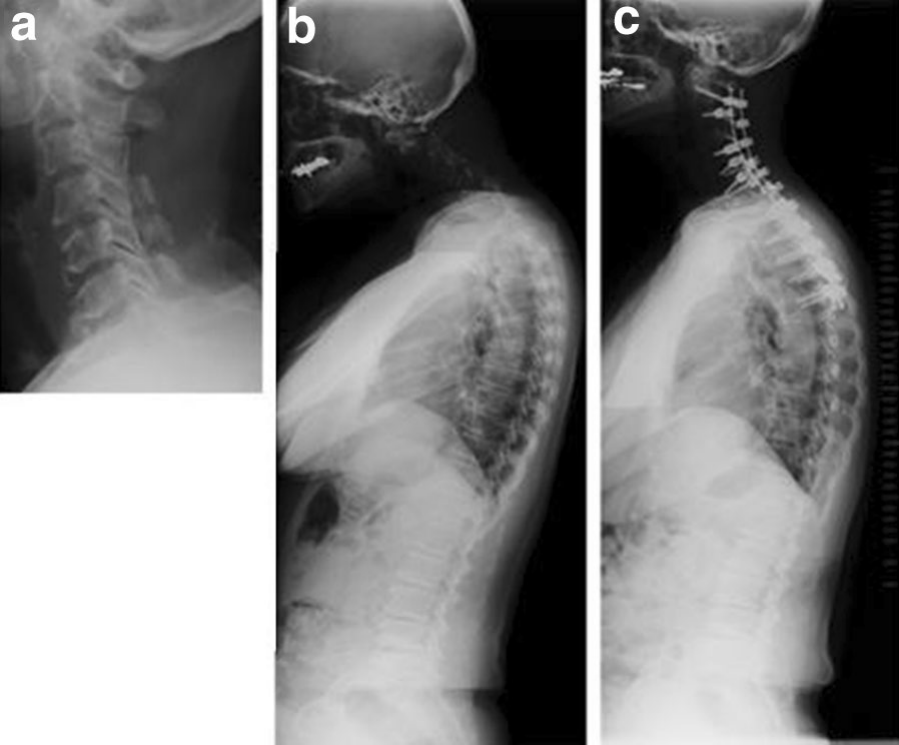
Case 1 radiographs. a Lateral plain radiograph of the cervical spine after laminoplasty for cervical spondylotic myelopathy showing normal sagittal alignment. The patient complained of head drop and severe low back and lower extremity pain 2 months after laminoplasty. b Lateral whole-spine radiograph showing marked cervical spine kyphosis and lumbar spine hyperlordosis. c After cervical corrective fusion (C2–C4), cervical kyphosis and lumbar hyperlordosis, as well as lumbar spine symptoms, were relieved. *C* cervical vertebra
